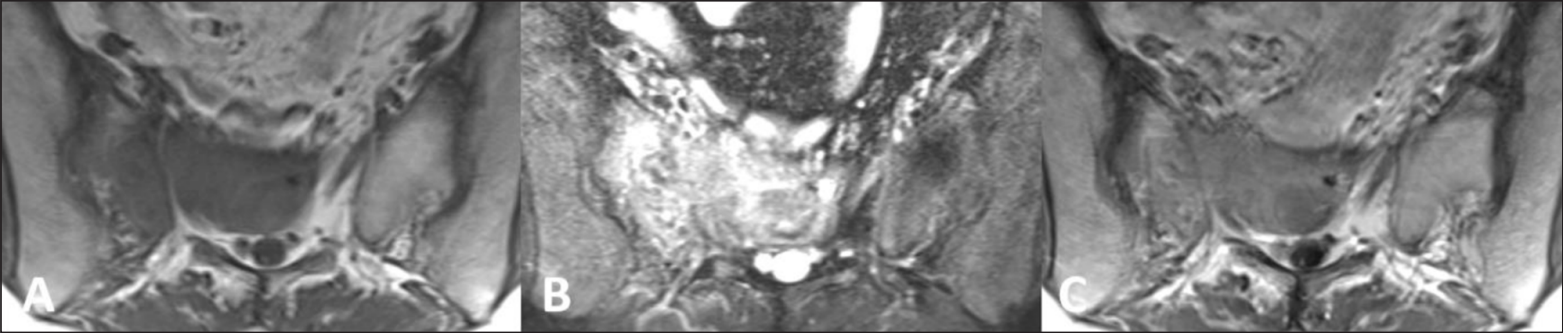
A 40-year-old female with giant cell tumour of the sacrum. (a) Axial T1-weighted MR image shows a homogeneous hypointense mass located on both sides of the midline with extension into the presacral soft tissues. (b) Axial fat saturated T2-weighted MR image shows heterogeneous high signal intensity. (c) Axial post-contrast T1-weighted MR image shows moderate enhancement.
